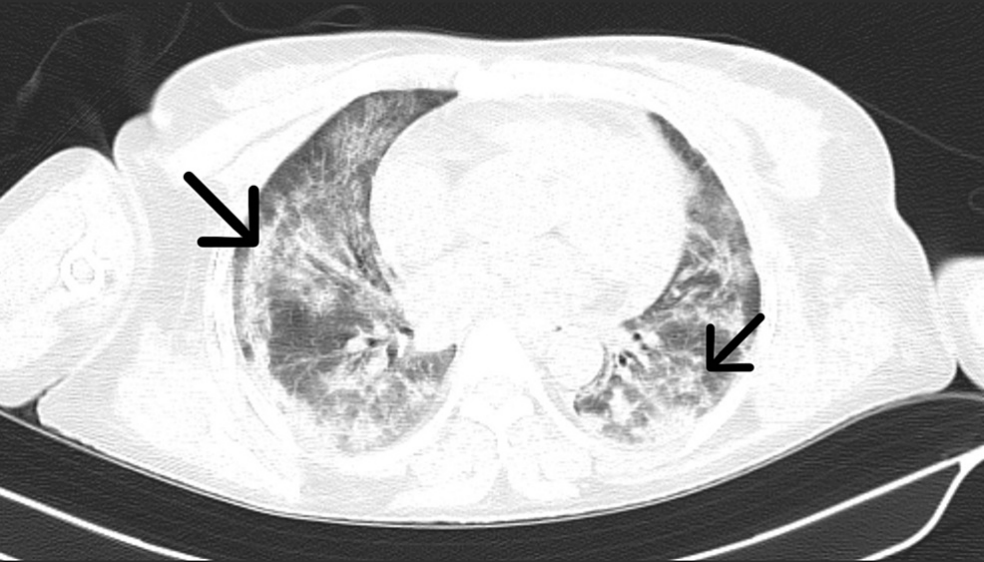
High-resolution computed tomography (HRCT) of the thorax (axial view) Black arrows show ground glass opacities in the lungs
